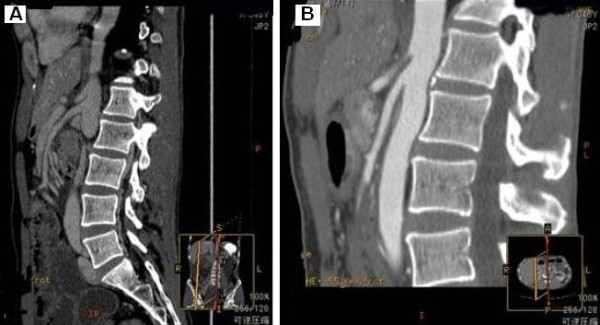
Reconstructed enhanced multidetector-row computed tomography reveals the following:(A) before the surgical repair of the right paraduodenal hernia, the aorto-mesenteric angle is 44°, and the aorto-mesenteric distance is 28 mm. There are intestinal gas bubbles under the aorto-mesenteric junction. (B) After the repair, the aorto-mesenteric angle has narrowed to 14°, and the aorto-mesenteric distance has shortened to 5 mm. Furthermore, no intestinal gas can be seen under the angle.
